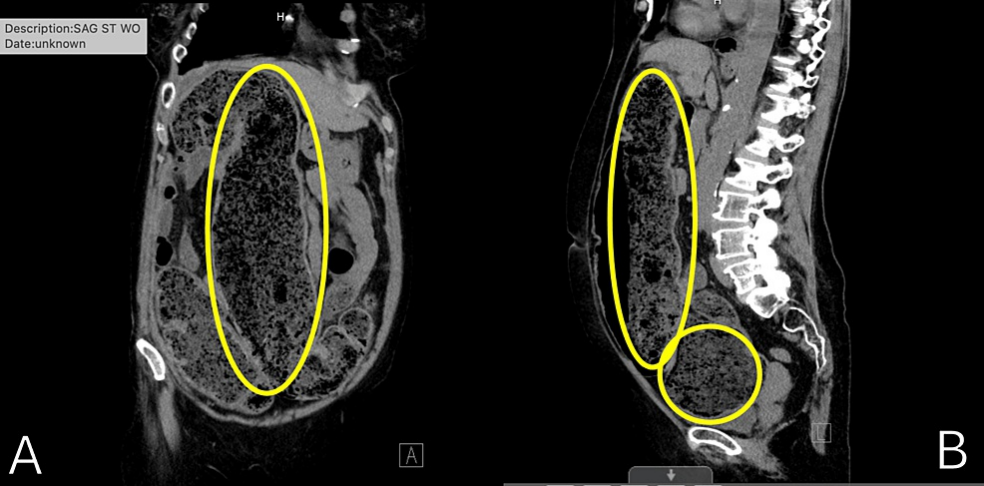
(A) Coronal CT of the abdomen showing colonic dilatation of the ascending and transverse colon. (B) Sagittal CT of the abdomen revealing colonic dilation of the transverse colon
